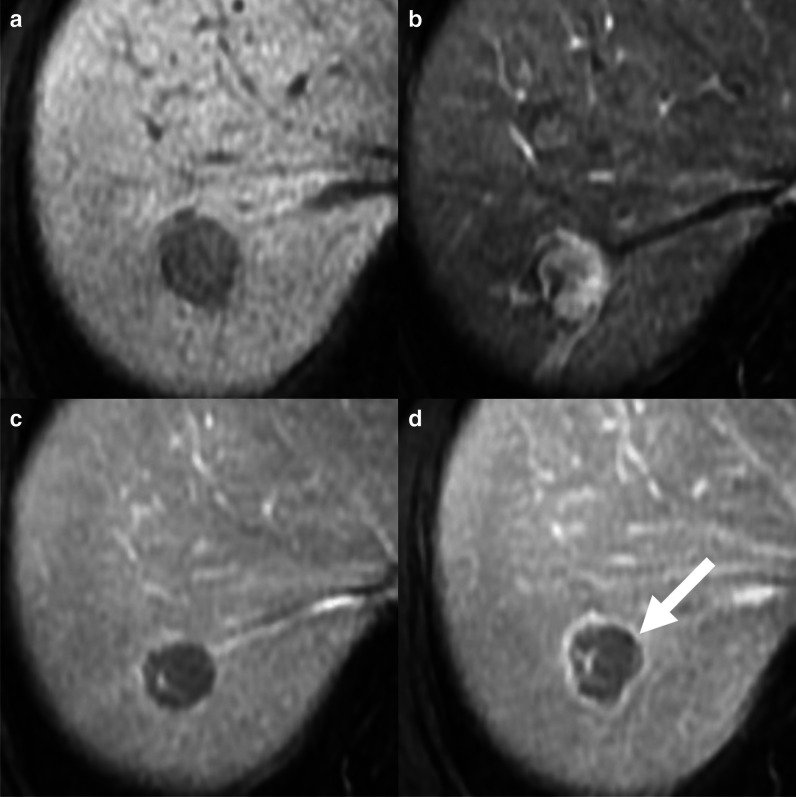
A 66-year-old man with cirrhosis and hepatocellular carcinoma. MRI acquired after the administration of extracellular contrast agent shows a 20-mm lesion with hypointensity on pre-contrast image (a), non-rim arterial phase hyperenhancement (b), non-peripheral washout and enhancing capsule on portal venous (c) and delayed (d, arrow) phases
